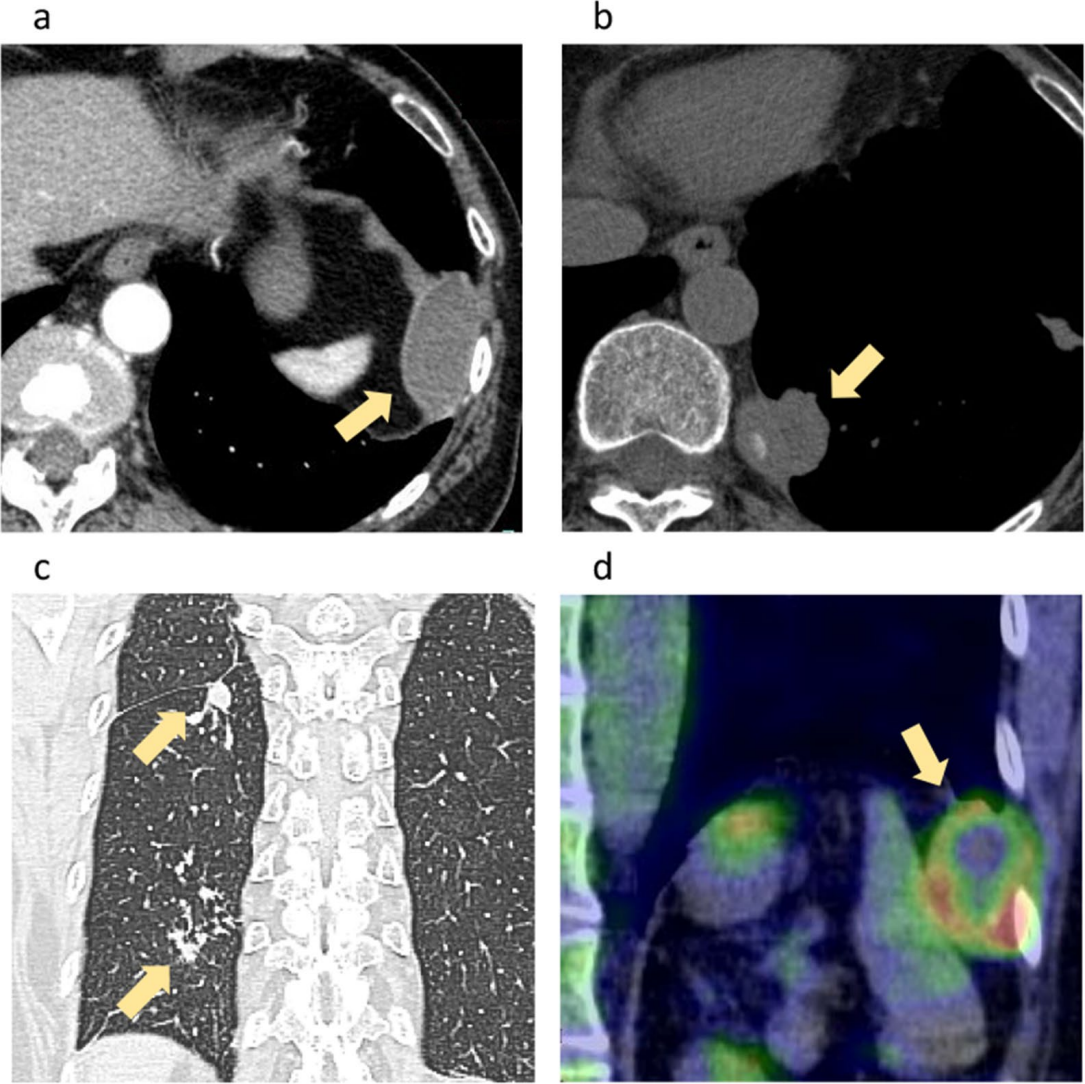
CT and PET–CT. High-resolution lung CT images showed (a) a cystic lesion in the left diaphragm, b focal thickening of the posterior mediastinal pleura with calcification, and c multiple small nodules in both lung fields. d PET–CT (coronal view) showed increased uptake of fluorodeoxyglucose in the cystic lesion. CT, computed tomography; PET–CT, positron emission tomography–computed tomography
